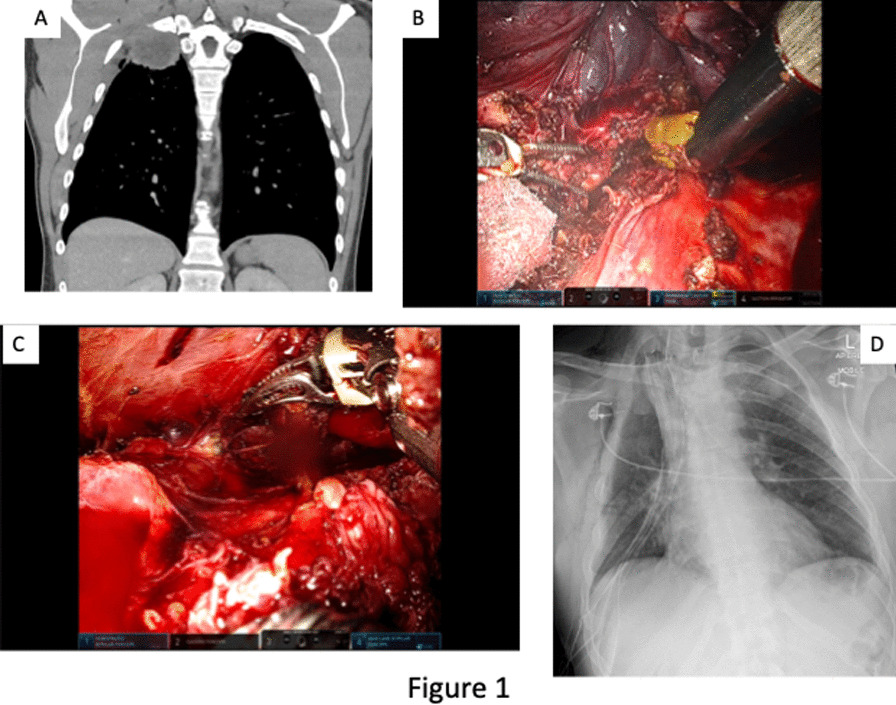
A Right upper lobe tumour invading the chest wall, B Anterior chest wall dissection from outside towards the apex, where Brachial plexus was preserved, C Posterior chest wall dissection and disseconnection of ribheads with transverse processes, D Post operative Chest Xray after resection
